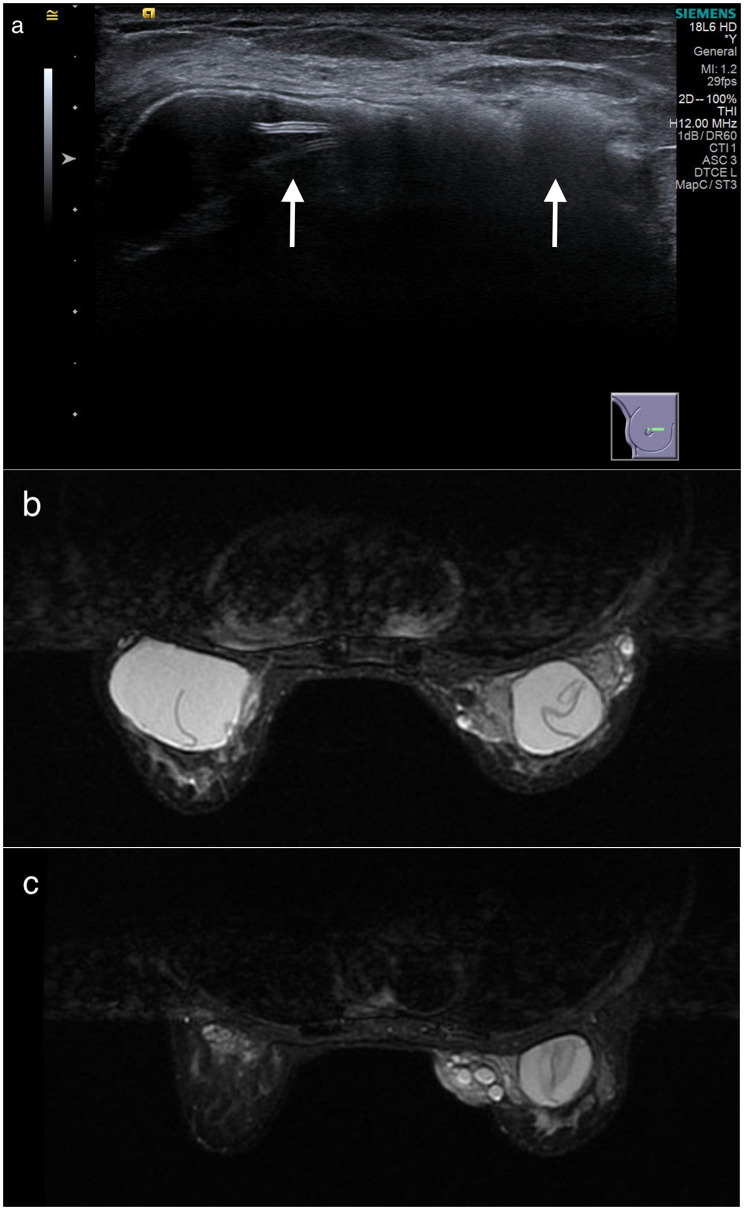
Diagnosis based on breast sonography and MRI. (a) Breast sonography shows intracapsular rupture of the implant. We observed a loss of the integrity of the shell with its fragments inside the implant (stepladder sign, left arrow). We observed an extracapsular rupture of the implant. The evident snowstorm pattern (right arrow) of the silicone outside the shell is characteristic of silicone outside the implant. (b) In MRI findings, the ‘linguine sign’ represents intracapsular rupture. (c) MRI shows that the ruptured implant migrated freely beyond the envelope and the fibrous capsule into the surrounding breast tissue. MRI, magnetic resonance imaging
